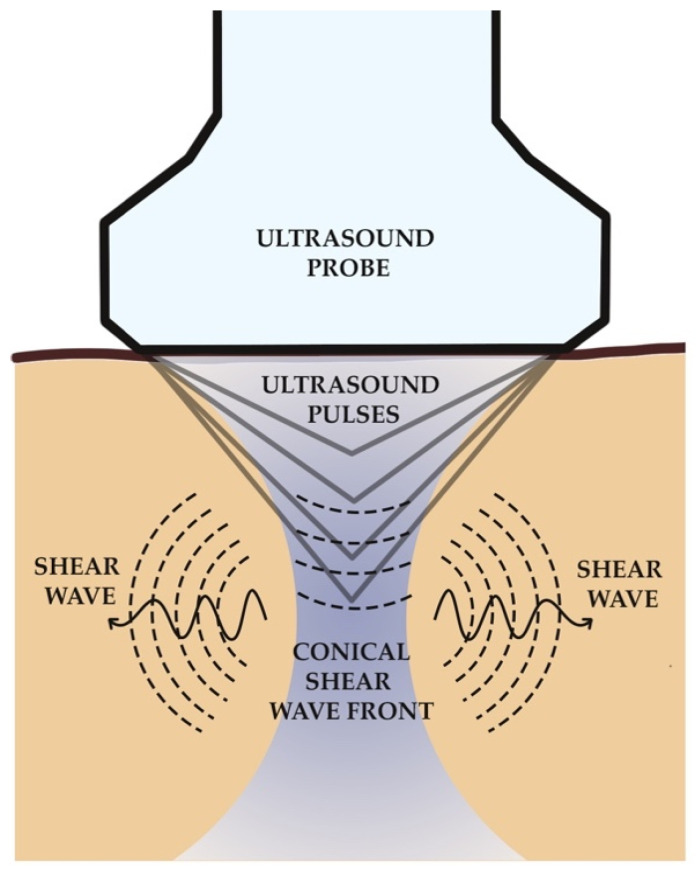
Basic principle of shear wave elastography (SWE). Ultrasound probe produces a localised radiation force deep in the tissue of interest, inducing a shear wave that propagates from this focal point. Numerous focal points are generated simultaneously in a line perpendicular to the skin surface. A conical shear wave front is created that sweeps the image plane on each side of the focal point. Adapted from Sigrist et al. [54] and Early et al. [55].
